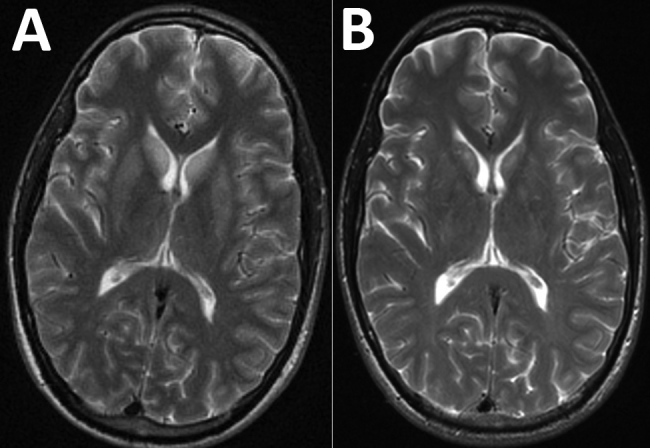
Magnetic resonance imaging (MRI) of the brain of a patient with encephalitis caused by Powassan virus, Massachusetts, USA, 2017. A) Initial brain MRI showing high T2 signal abnormality in the bilateral caudate and putamen. B) Noticeable improvement on repeat brain MRI 2 weeks later.
